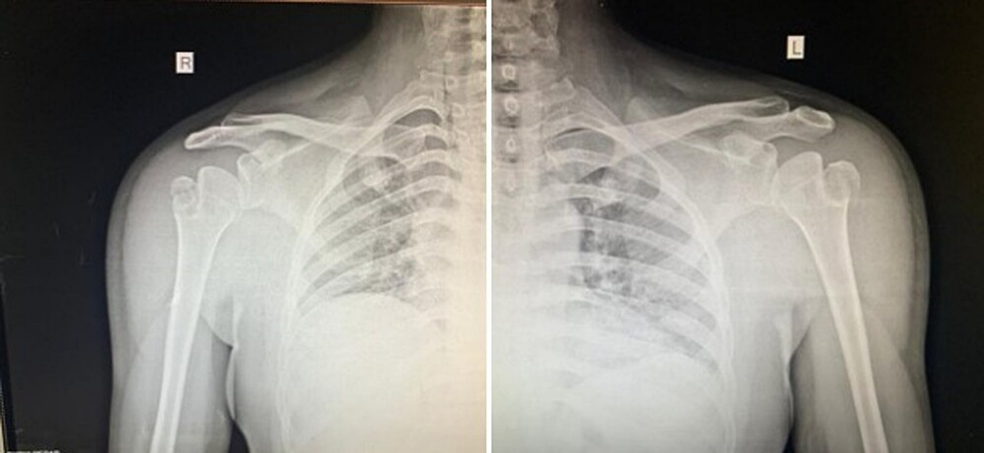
Post-reduction radiographs of bilateral shoulders
